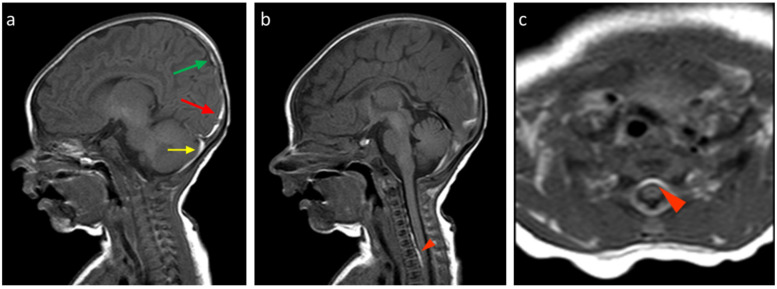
Birth-related SDH in a 9-day-old infant born spontaneously: (a) Right paramedian sagittal plane T1-weighted SE sequence—the presence of subdural hematomas with occipital (red arrow), parietal (green arrow), and cerebellar (yellow arrow) distribution. (b) Midsagittal plane T1-weighted SE sequence—similar finding in the spinal canal anteriorly in the cervico-dorsal tract (arrowhead). (c) SE T1-weighted sequence in the axial plane passing through the distal cervical tract—confirmation (arrowhead).
